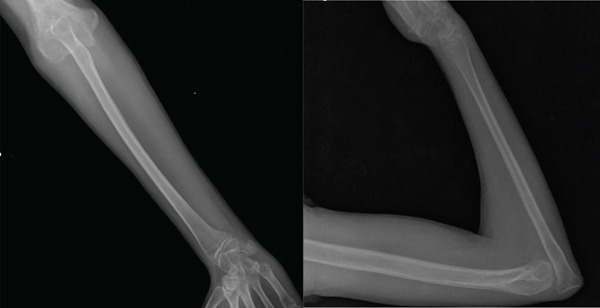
Postoperative radiographs of the right forearm demonstrating complete ulnar resection with preservation of the elbow joint.
